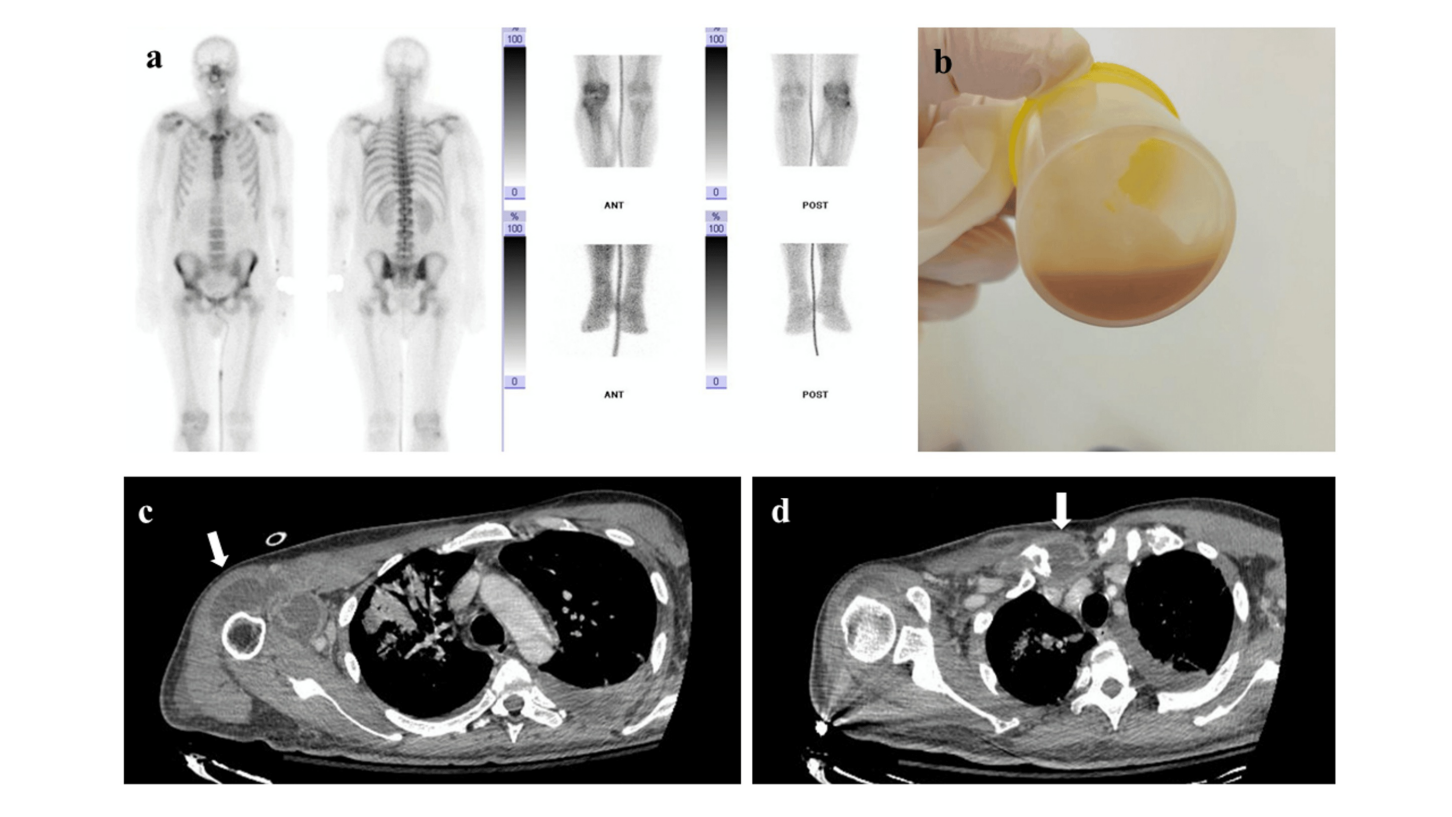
Bone scintigraphy showing polyarthritis involving the sternoclavicular joints, the right shoulder, and the right knee joint (a). Pus aspirated from the right shoulder (b). CT scan showing bursitis of the right shoulder (white arrow) (c). CT scan showing bursitis of the right sternoclavicular joint (white arrow) (d).
